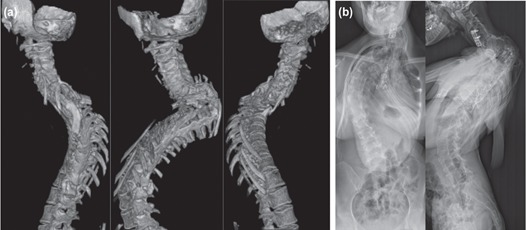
(a) Computerised tomography images showing fusion of the instrumented level. (b) Radiographs of the whole spine showing no further progression of spinal deformity.
